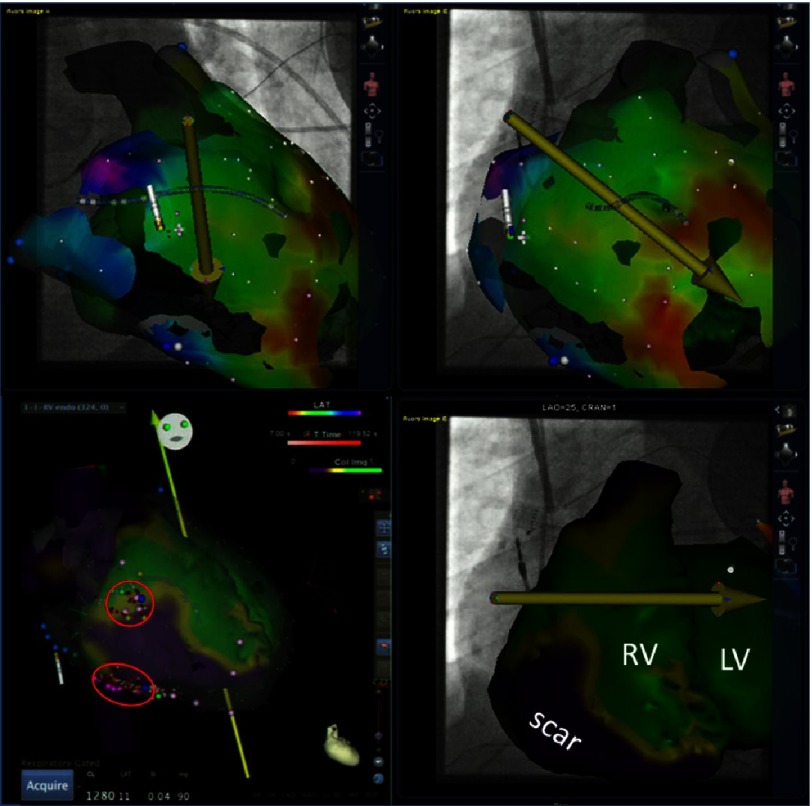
Top panels: Depiction of the epicardial mapping process using remote magnetic navigation. The catheter is moved in the epicardial space by moving the yellow vector in various directions and thereby changing the direction of the catheter tip. A mechanical drive allows advancement and retraction of the catheter and thereby free accessibility of the entire epicardial space. Bottom panels show the image integration of the fused images from nuclear (perfusion) and CT 3D segmentation. Green colour depicts normal perfusion, while purple depicts perfusion scar and yellow the border zone. Please note the normal LV perfusion (LV –EF is 66%), but the large perfusion scar of the RV. Purple tags mark late ventricular potentials.
